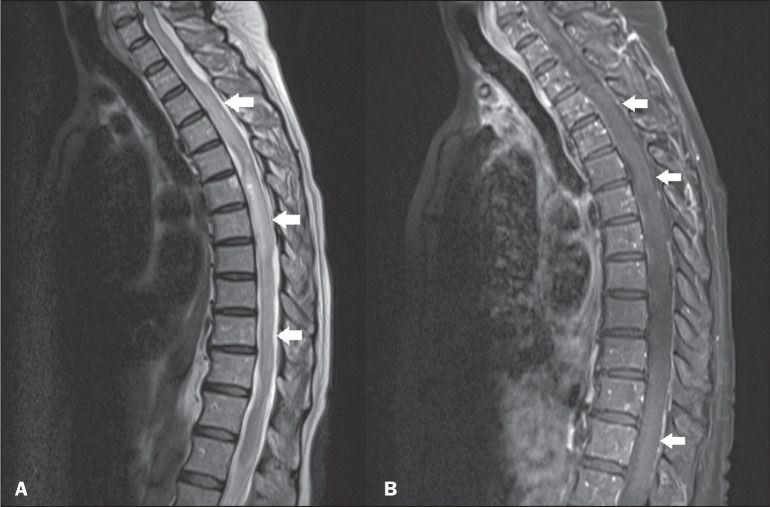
Lupus. Contrast-enhanced sagittal T2-weighted and T1-weighted MRI scans
